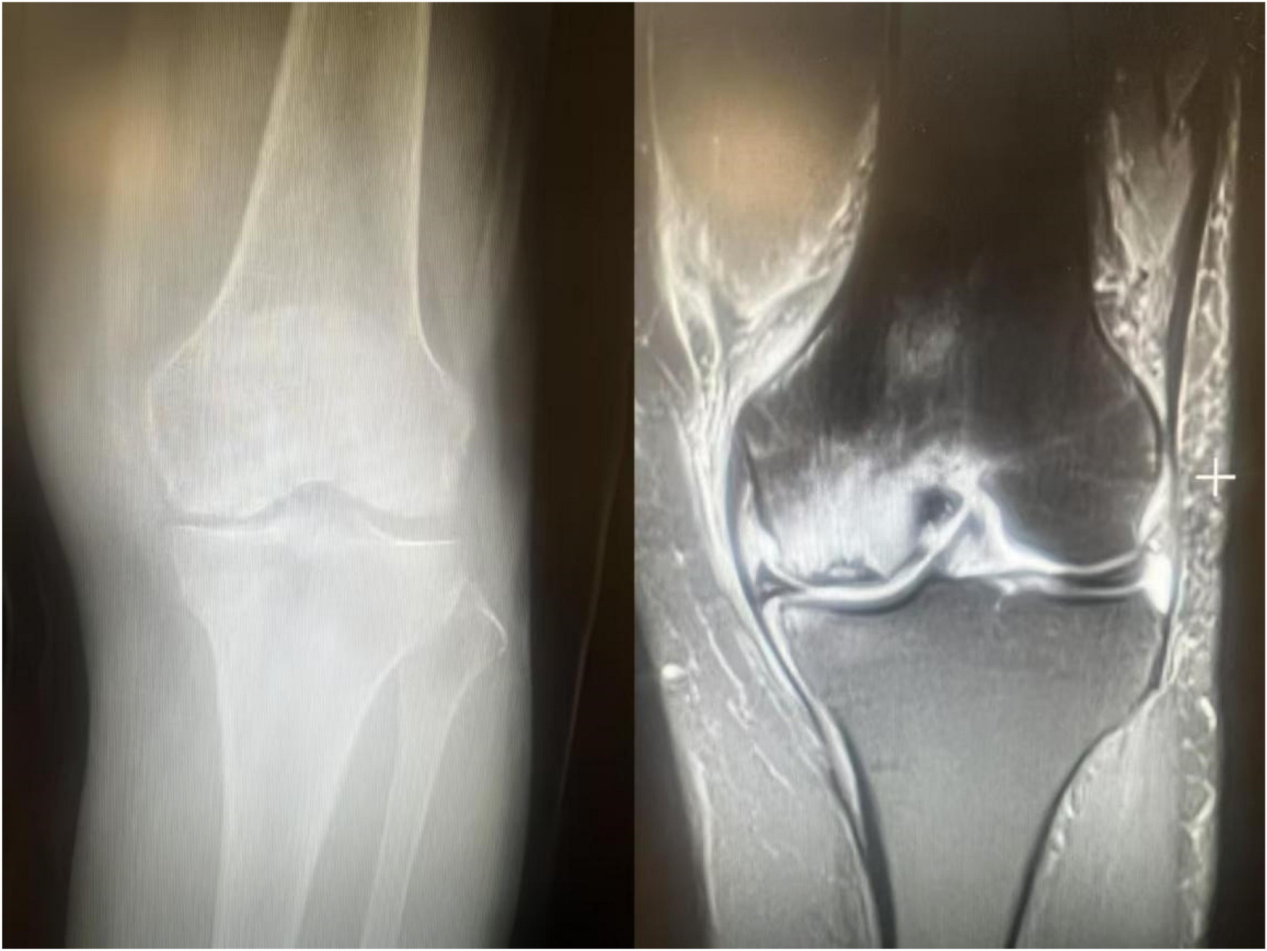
Coronal position of preoperative x-ray and MRI in patients with SIFK.
